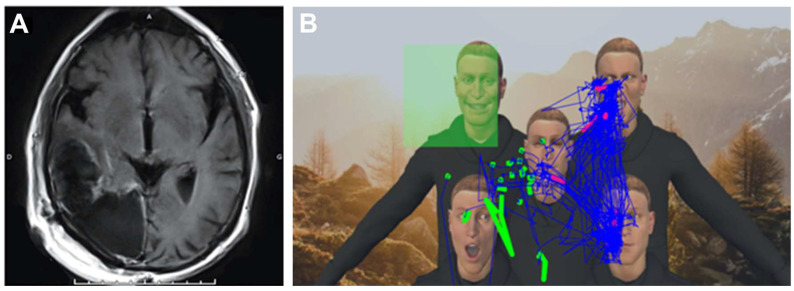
(A) Postoperative MRI scan for a patient with malignant right occipital glioma. The patient presented with homonymous hemianopia and USN. (B) Postoperative bedside VR-TANGO test: eye-tracking feedback for 10 cumulative tests. The patient was asked to determine the emotion expressed by the face looking at him (here, the upper left face, indicated by the green square). The eye tracking data recorded for the patient (blue lines) showed that he was unable to direct his attention to the left side. (USN, unilateral spatial neglect).
